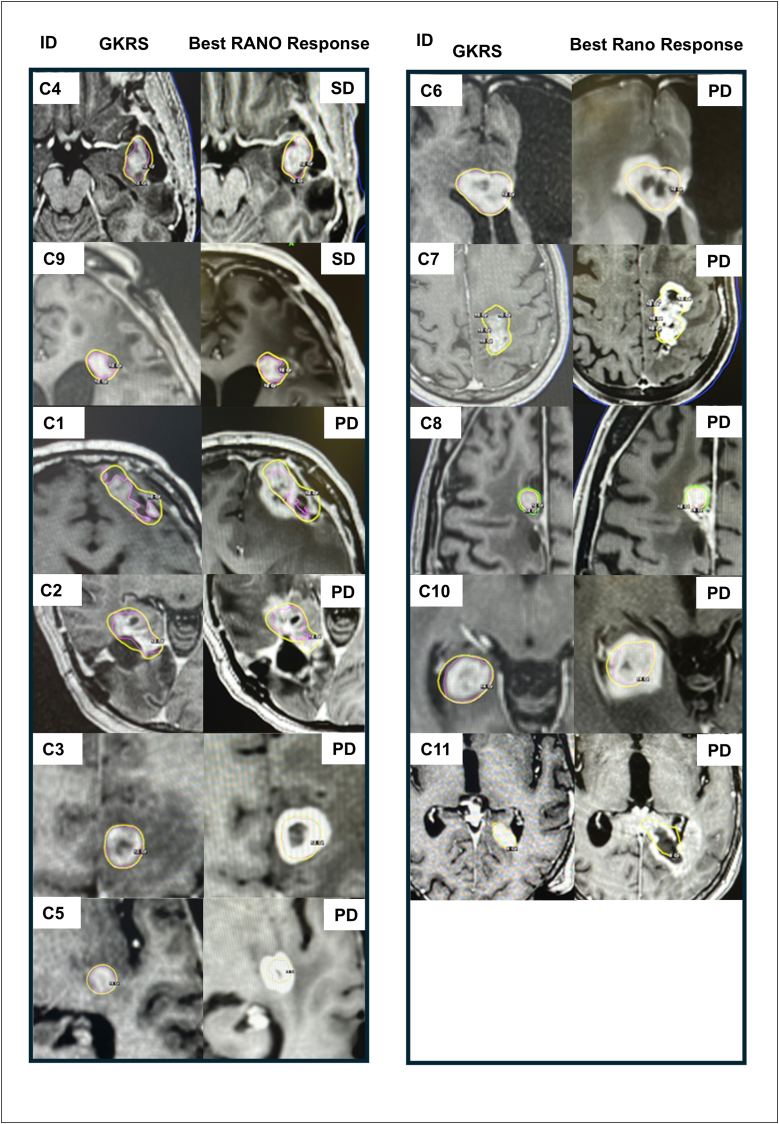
MRI at baseline and best RANO response during follow-up for 11 concurrent control patients C1–C11. GKRS, Gamma Knife radiosurgery; C, control; RANO, Response Assessment in Neuro-Oncology; CR, Complete response; PR, Partial response; MR, Minimal response; SD, Stable disease, PR, Progressive disease.
